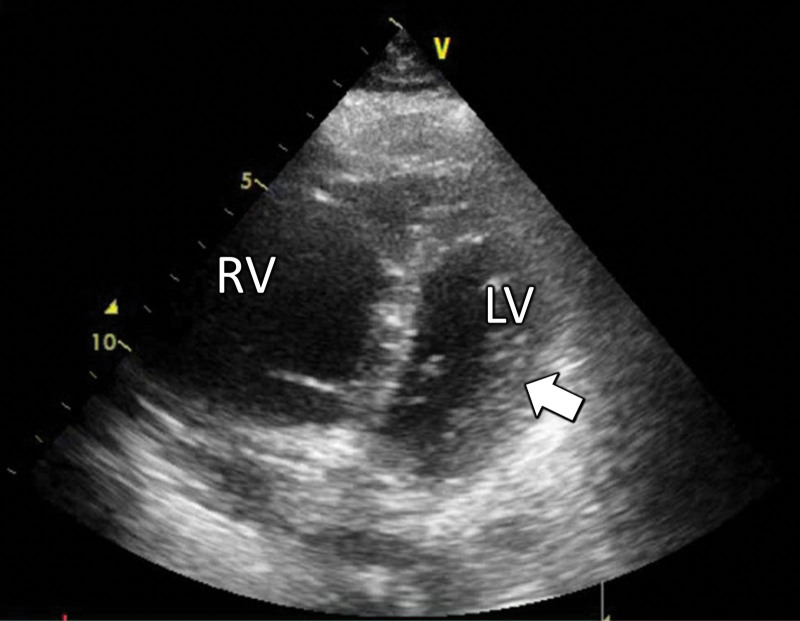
Parasternal short axis view on transthoracic echocardiography showing the marked enlargement of the right ventricle and collapse of the left ventricle (white arrow). LV = left ventricle, RV = right ventricle.
